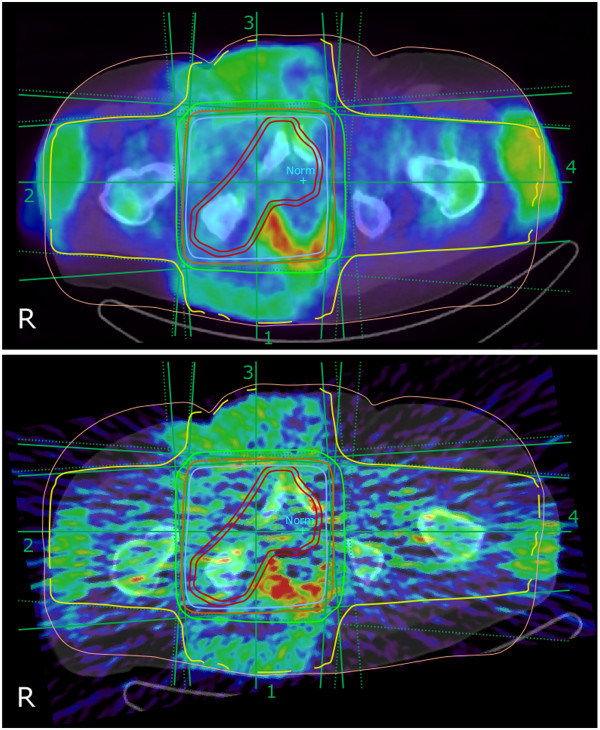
Transaxial overlaid 50-MV radiation treatment plan on PET/CT (0 to 30 min) image. This is for three-dimensional ordered subset expectation minimization (3D-OSEM, upper) and filtered back projection (FBP, lower) reconstruction. The treatment, here shown for plane section through the normalization point (Norm), is a four-field box technique with the target dose delivery of 8 Gy in total divided equally between the four beam portals. The isodose curves displayed cover the ranges 35% (yellow), 65% (green), 85% (orange), and 95% (blue) of the total dose. The two red curves depict the interactively defined clinical target volume (inner line) and planning target volume (outer line). The gross tumor volume is not depicted but includes the prostate together with the metastasis of the right os ischii and the medial portion of the right os pubis.
